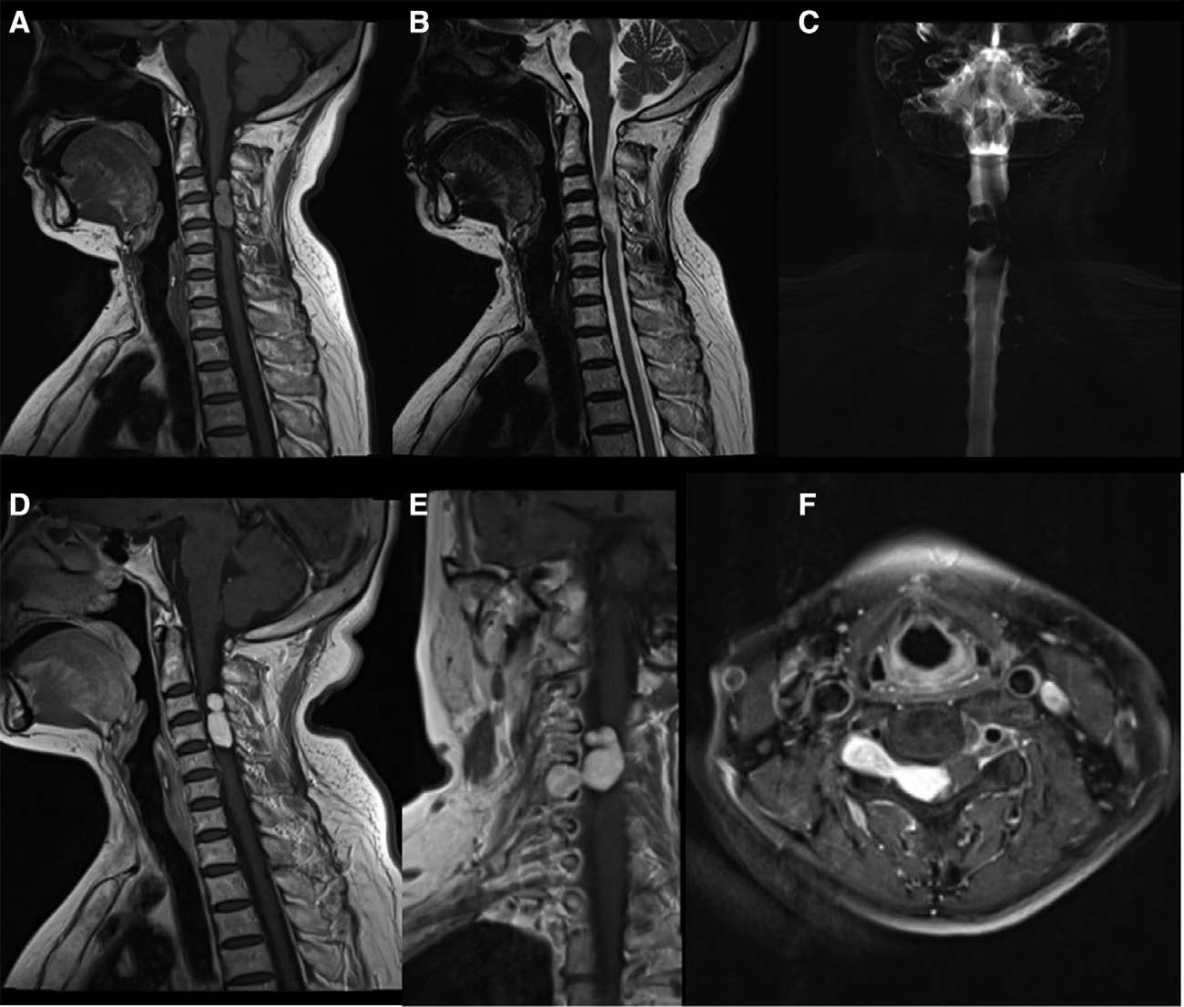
MRI of the cervical spine in a 60-yr-old female. Views: (A) The tumor was located at the level of cervical vertebrae 3 to 5 and appeared with high signal intensity on sagittal non-contrast T1WI. (B) The tumor showed uneven high signal intensity and a slight low signal intensity on sagittal T2WI. (C) Magnetic resonance myelography showed that the mass pushed the spinal cord to the left, and the subarachnoid space at the upper and lower edges of the mass widened, suggesting that the mass was located under the dura, not outside the dura. (D) The tumor is significantly enhanced, showing extremely high signal intensity on contrast-enhanced sagittal T1WI, although the tumor showed high signal intensity on non-contrast T1WI. (E) The tumor is dumbbell-shaped and located inside and outside the spinal canal, with the thinnest part located in the intervertebral foramen on coronal contrast-enhanced T1WI. (F) The tumor is dumbbell-shaped and hyperintense on contrast-enhanced axial fat-suppressed T1WI. MRI = magnetic resonance imaging, T1WI = T1-weighted imaging, T2WI = T2-weighted imaging.
